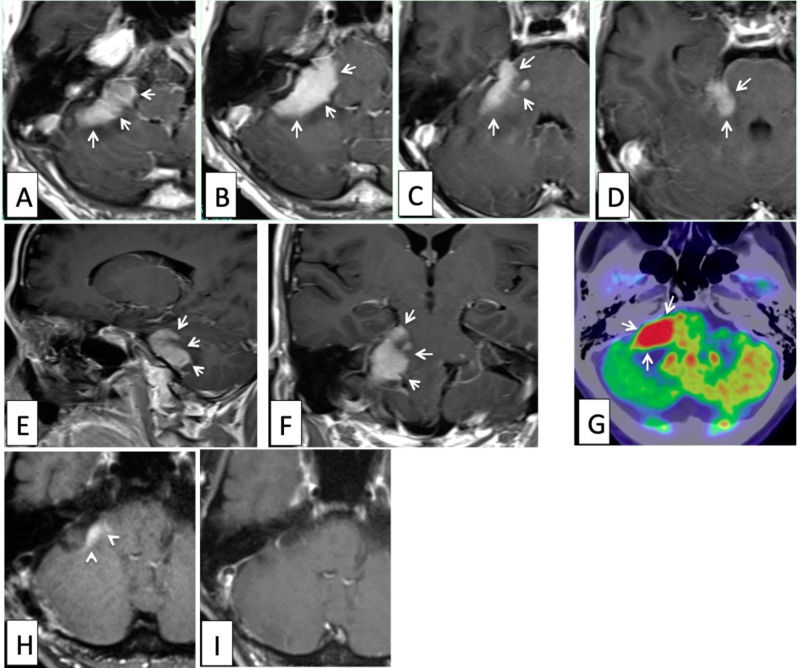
Magnetic resonance imaging (MRI) demonstrating a well-enhanced mass lesion in the right cerebellopontine (CP) angle appearing to have originated in the cerebellum Axial (A, B, C, D), sagittal (E), and coronal (F) images of gadolinium (Gd)-enhanced T1-weighted MRIs at presentation. A well-enhanced tumor was located mainly in the right cerebellopontine angle brain parenchyma (white arrows). It showed high-uptake of fluorodeoxyglucose (FDG) on positron emission tomography (PET) (G, white arrows). Only a small part of the tumor remained on a postoperative axial image of the Gd-enhanced MRI (H, white arrowheads). No enhanced mass was seen on an axial image of the MRI (I) after chemotherapy using high-dose methotrexate (MTX).
